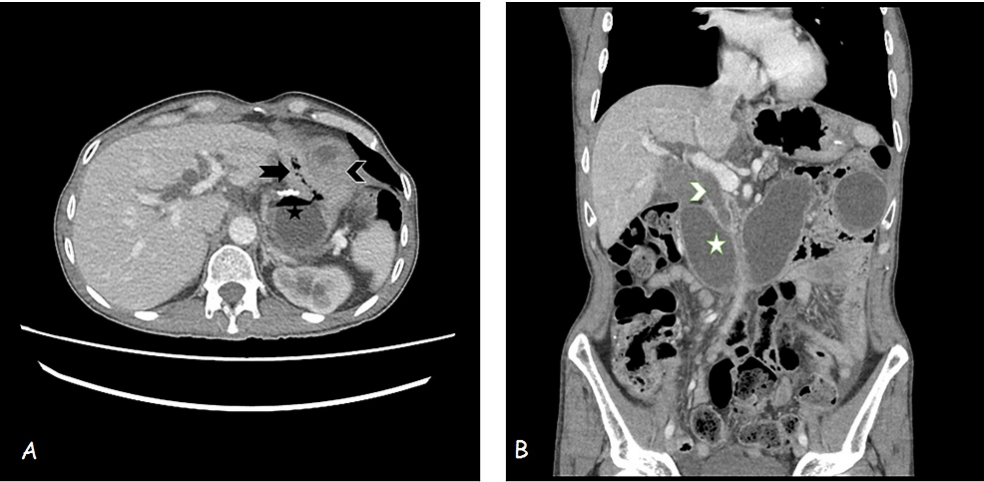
Contrast Enhanced CT Abdomen and Pelvis, Axial (A) and Coronal (B) Sections A - Axial section showing subtotal gastrectomy (black star) and loop gastro-jejunostomy. The efferent loop anastomotic site appears patent (black arrow) but the afferent loop anastomotic site shows thickening (black arrowhead). B - Coronal section shows dilated afferent jejunal loop (white star) in mid-abdomen with common bile duct (CBD) seen opening into that loop (white arrowhead). CBD is also appearing dilated with dilated intrahepatic biliary channels as well.
